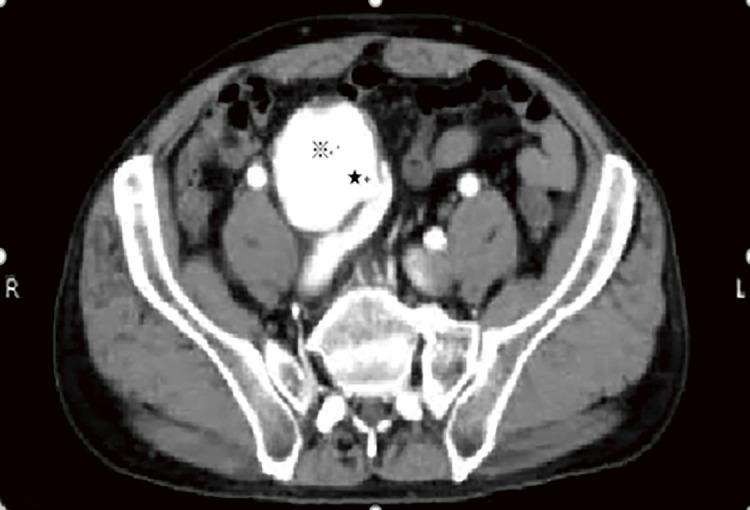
Early-phase contrast-enhanced computed tomography is shown. Contrast-enhanced computed tomography reveals a 60-mm-in-diameter right CIAA and contrast effects (※), from the right CIA to the right CIV (★), confirming the diagnosis of an IIAVF. The IVC runs anterior to the right CIAA and is dilated at the proximal aspect. Because contrast medium outflow into the CIV has been observed in the early phase, a large shunt is thought to have formed. CIAA, common iliac artery aneurysm; CIV, common iliac vein; CIA, common iliac artery; IVC, inferior vena cava; IIAVF, ilio-iliac arteriovenous fistula
